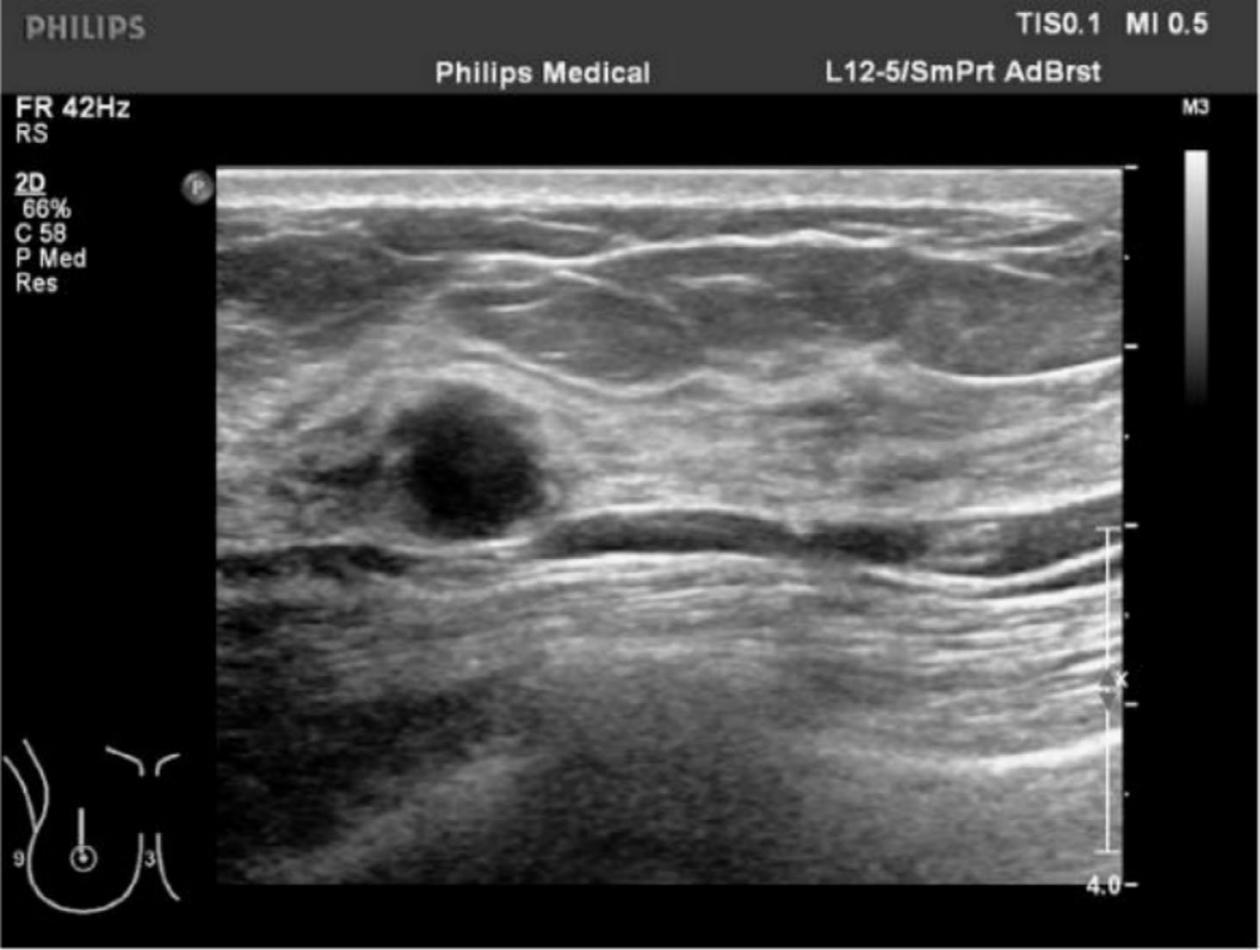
Ultrasound findings: A hypoechoic nodule in the right breast (BI‐RADS category 4A).
